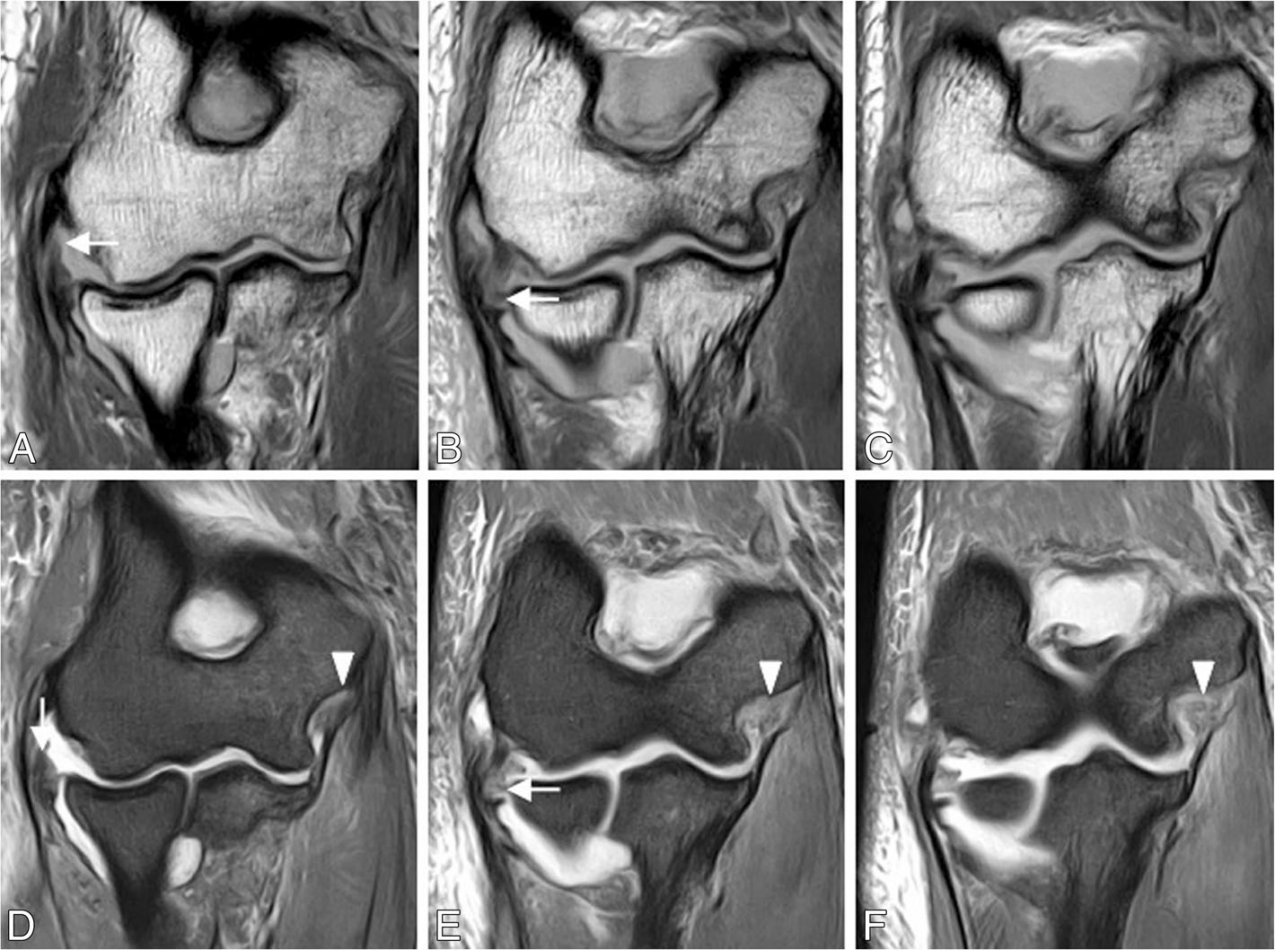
Posterolateral rotatory instability, stage 3B. Consecutive coronal T1-weighted MRI (a–c), and consecutive coronal FS PD-weighted MRI (d–f) showing an acute proximal full-thickness tear of the lateral ulnar collateral ligament and radial collateral ligament (white arrows) and anterior bundle of the medial collateral ligament complex sprain (white arrowheads)
